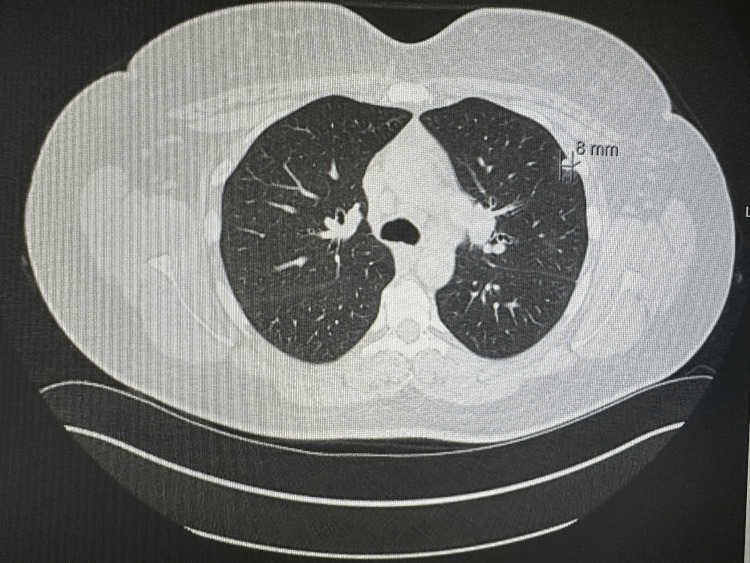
Computed tomography of the chest showing a pulmonary nodule in the left lung of 8 mm in size.
